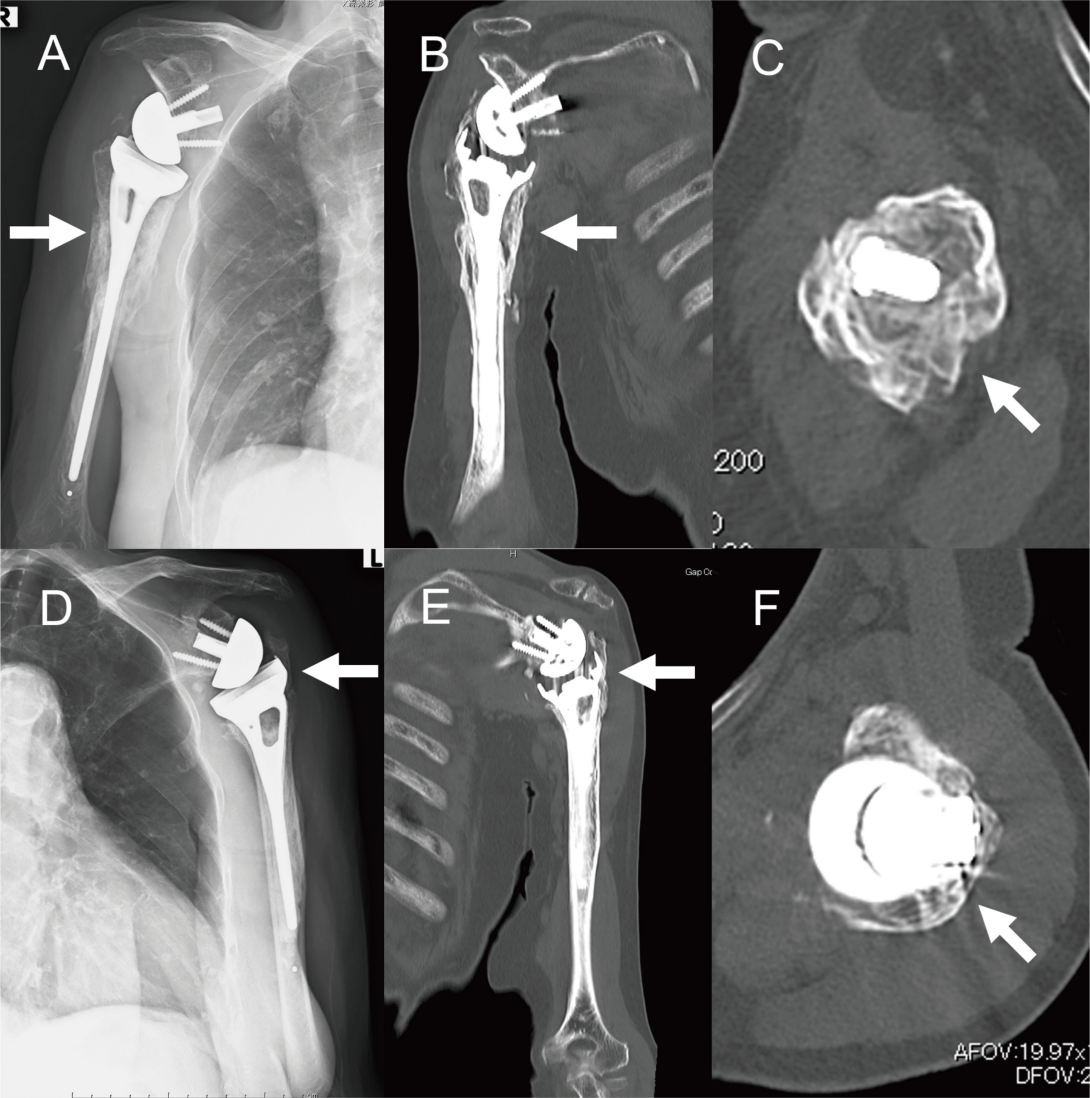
The final follow-up images. The shoulder images of left anteroposterior X-ray (A), coronal view of CT (B), axial view of CT (C), right anteroposterior X-ray (D), coronal view of CT (E), and axial view of CT (F). The fragments of both shoulders were ideally healed (indicated by arrows).
